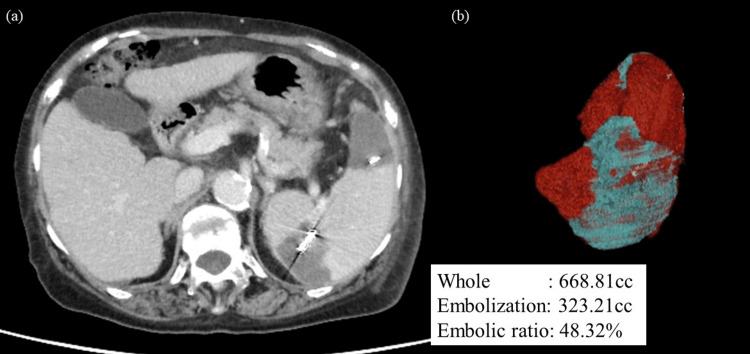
Computed tomography images showing changes in the spleen after partial splenic embolization (a) shows the effects of partial splenic embolization. The upper and middle portions of the spleen were embolized. (b) shows the spleen embolization rate. Approximately 48% of the spleen was embolized.
